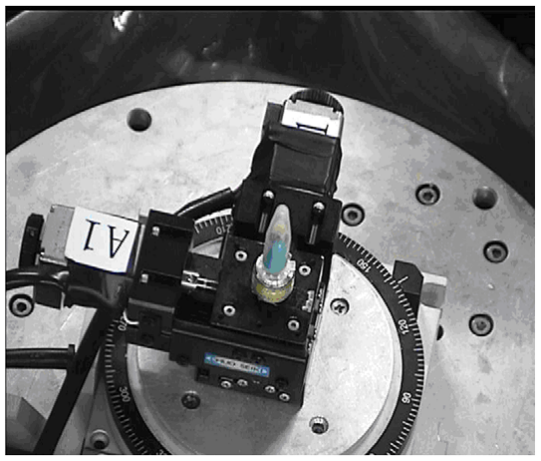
The X-ray ILPCI partial facility of SSRF. This is sample table. The sample can be rotated 180 degrees and is placed in the center. The sample has been wrapped in insulating material.
